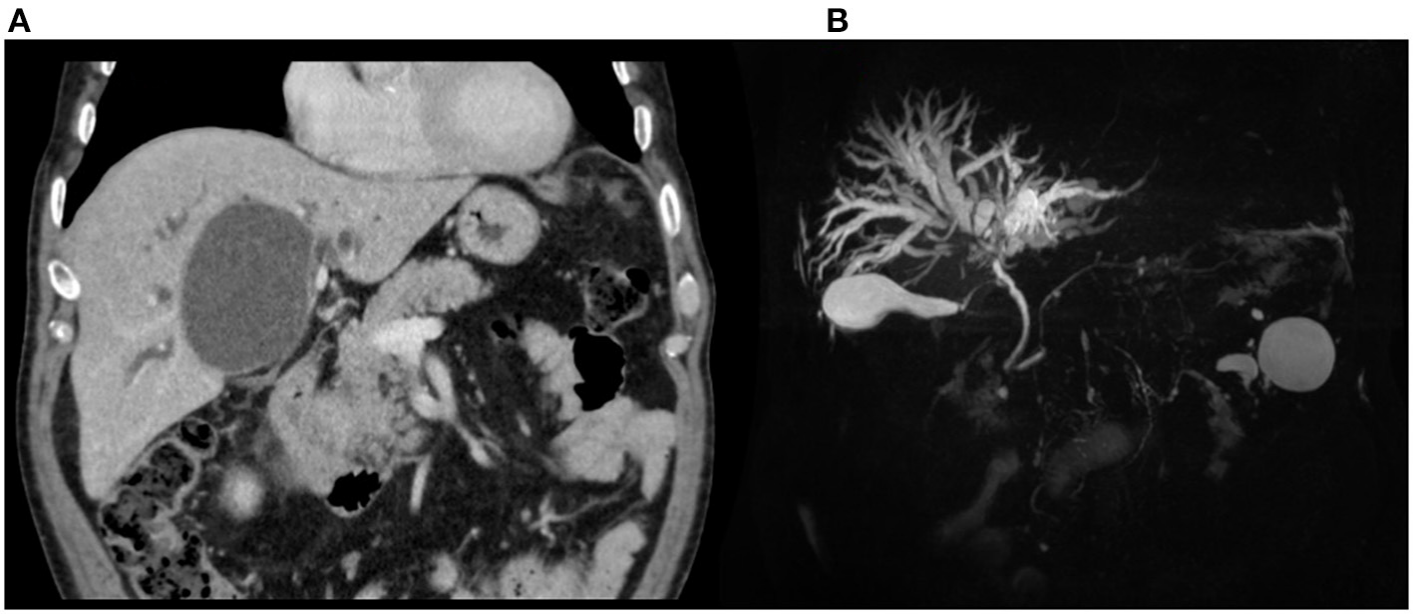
Patient 2, preoperative imaging: (A) CT scan and (B) MRCP demonstrating biliary confluence compression with dilatation.
